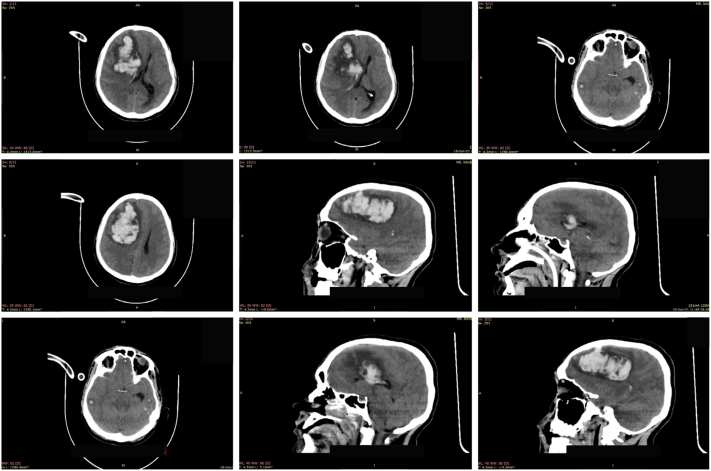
Non-contrast CT brain scan showing a large (8.7x5.7 × 4.2 cm) intraparenchymal hemorrhage seen in the right frontoparietal lobe with an extension of hemorrhage into the right lateral and 3rd ventricles, midline shift of 17 mm towards the left side, and effacement of suprasellar cisterns. In addition to focal hemorrhages in bilateral and temporal lobes, a small sulcal subarachnoid hemorrhage in the right temporoparietal lobe.
